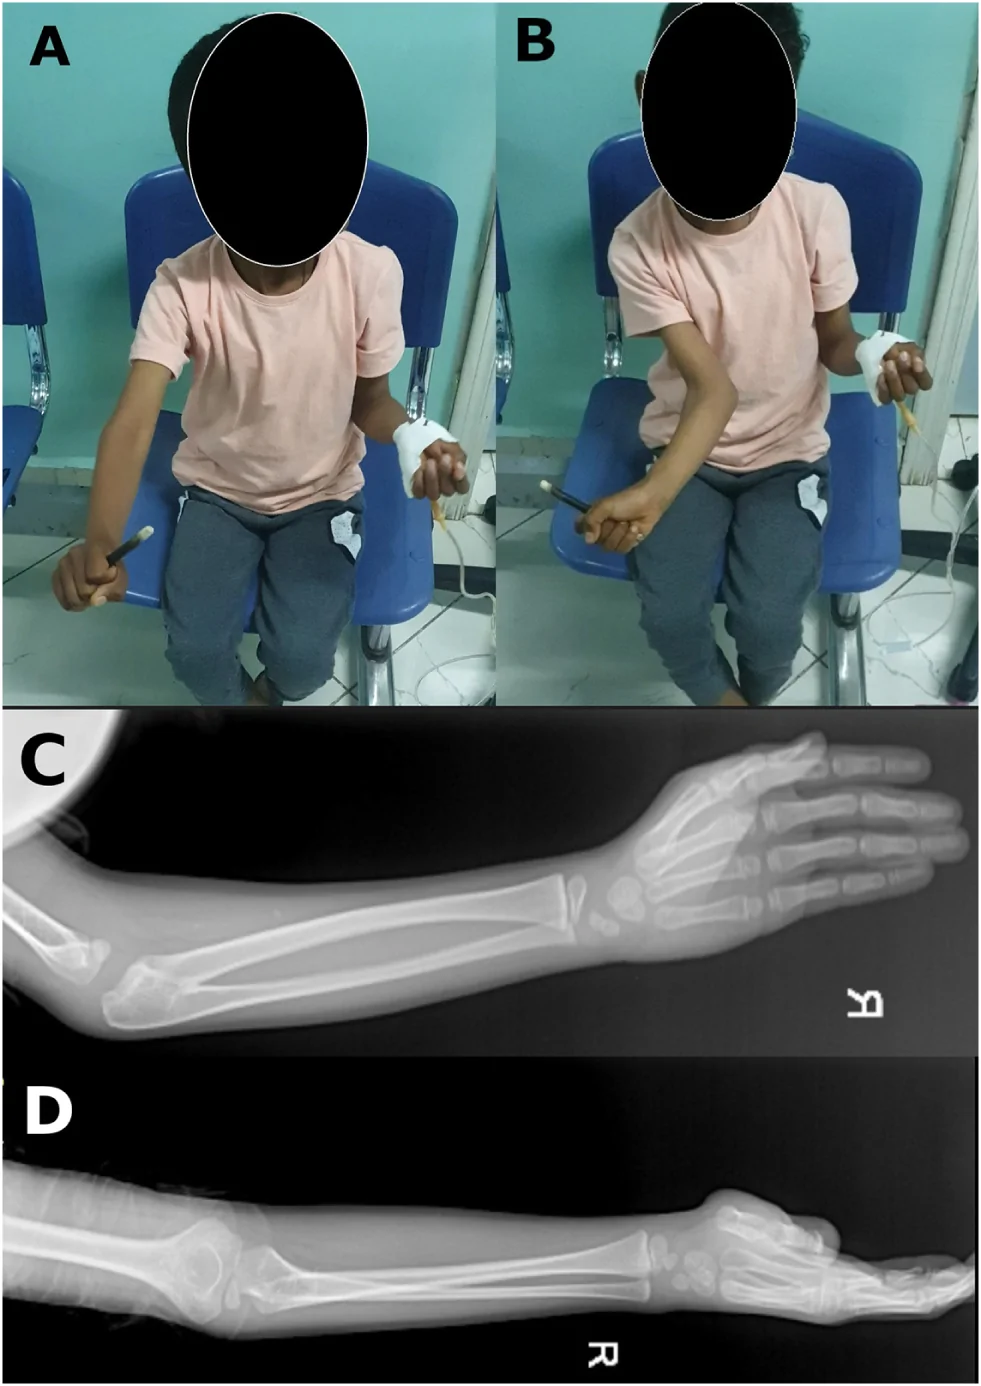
Preoperative findings. (A) Clinical view demonstrating the right forearm locked in fixed pronation. (B) Attempted supination revealing compensatory shoulder adduction and external rotation. (C) Anteroposterior radiograph showing osseous fusion at the proximal radioulnar junction. (D) Lateral radiograph demonstrating an anteriorly dislocated, mushroom-shaped radial head consistent with Cleary-Omer Type IV.
